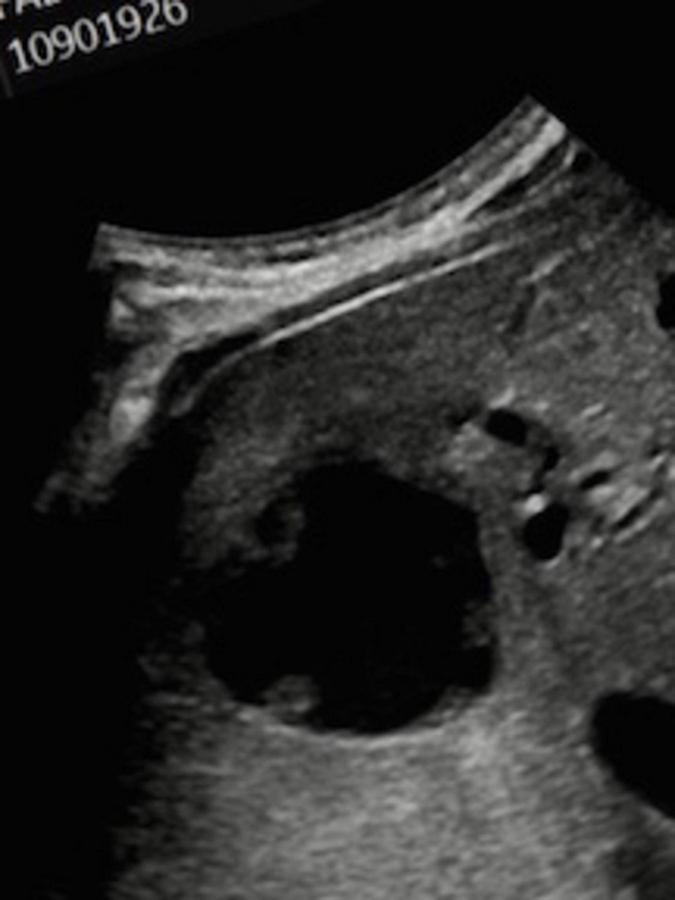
Ultrasound of liver showing an abscess. There is a mildly irregular, predominantly cystic lesion within the central hepatic parenchyma measuring 4.9 cm in largest dimension, new since a prior comparison ultrasound performed 8 months ago.
